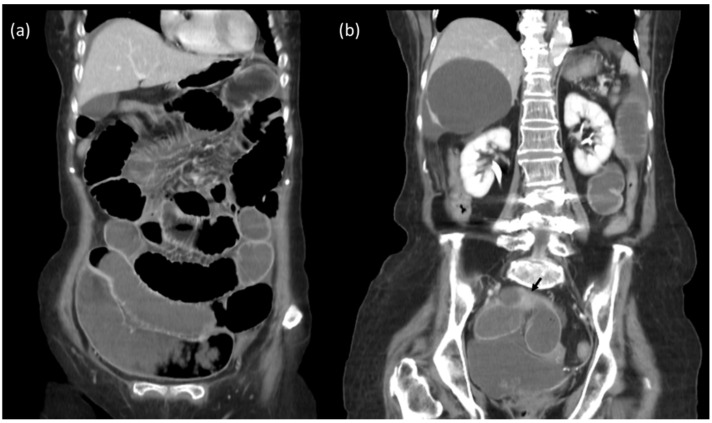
The 84-year-old female presented to emergency department having had abdominal fullness and constipation for 5 days. Initial KUB radiograph shows distended small bowels. Contrast-enhanced computed tomography of abdomen–pelvis: (a,b) Distended small bowel loops, with transition zone at ileum (black arrow). Mechanical obstruction due to adhesion was diagnosed.
